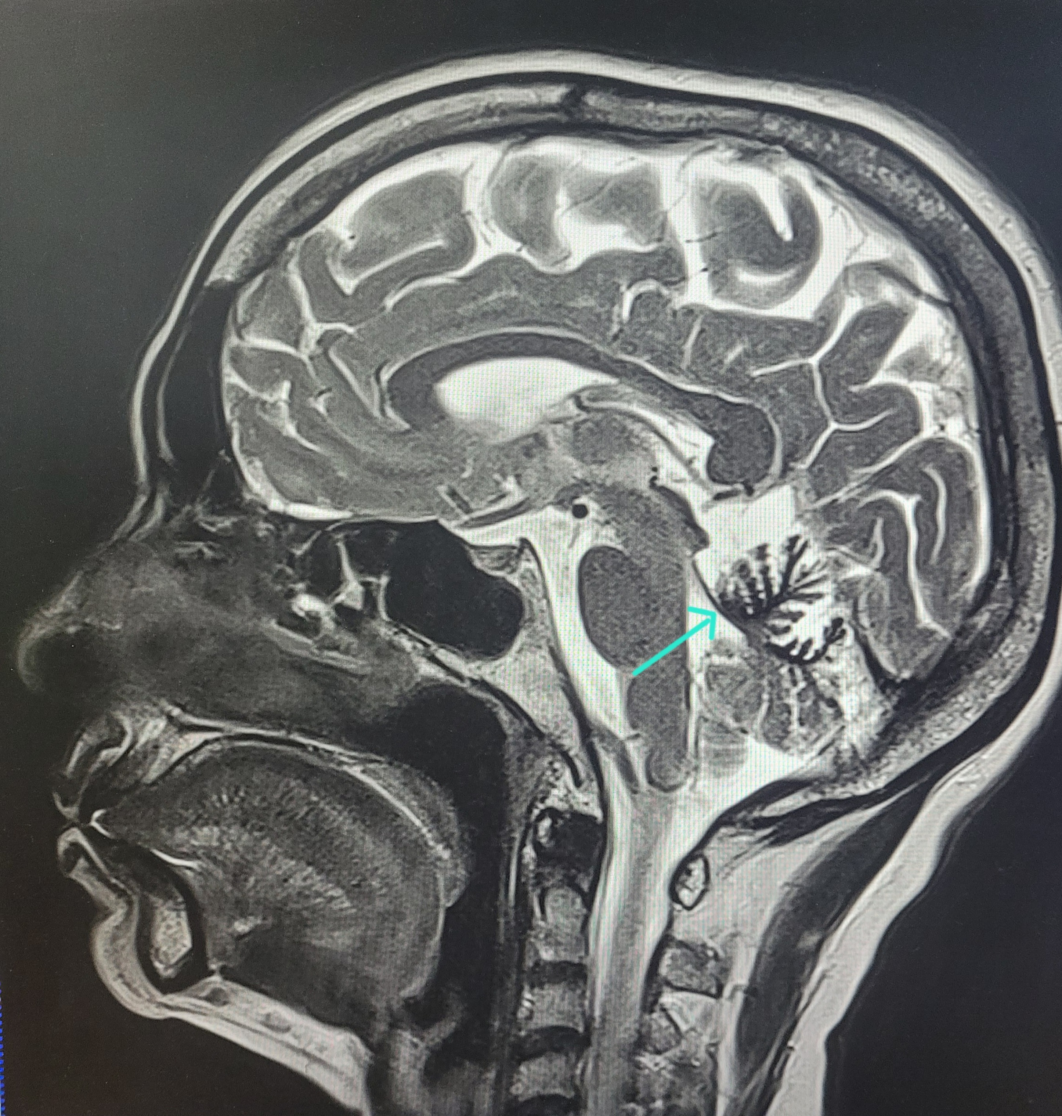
Mid-sagittal MRI showing iSS Type 1 T2-weighted mid-sagittal section MRI showing hypointensity along the medial aspect of vermis. iSS: infratentorial superficial siderosis; SWI: susceptibility-weighted imaging
